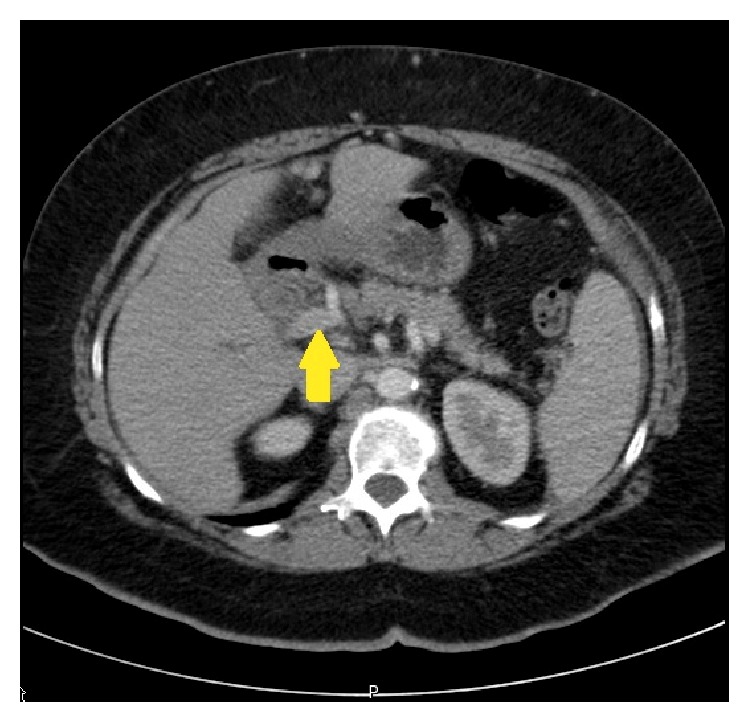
CT abdomen with and without contrast.
